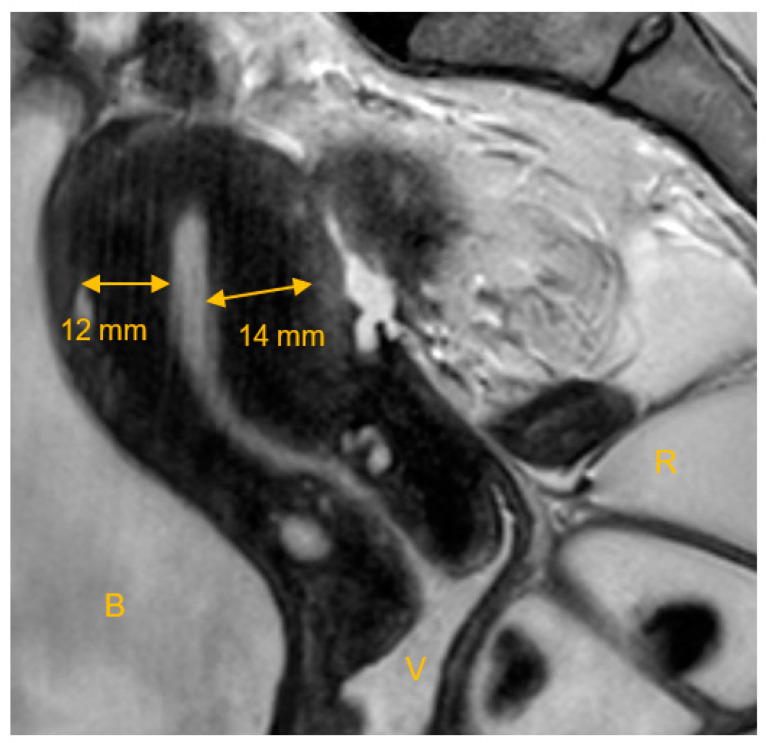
MRI characteristics of the uterine junctional zone. T2-weighted MRI in the sagittal plane, showing the widened junctional zone marked with arrows. B: bladder, V: vagina filled with ultrasound gel, R: rectum filled with ultrasound gel. Image acquisition on 3T, Ingenia Philips Healthcare, with a T2 TSE sequence.
